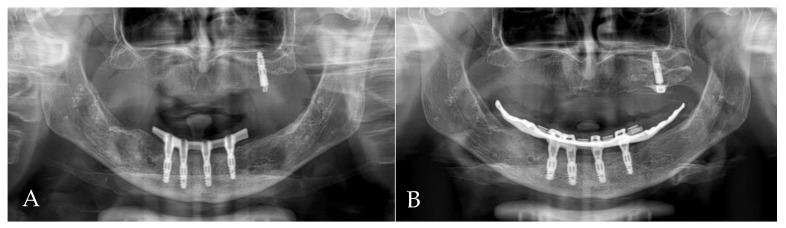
Orthopantomogram (OPG): (A) 4 months after first surgery—the prosthetic restoration’s finalization; (B) 24 months after bar-retained overdenture delivery.
